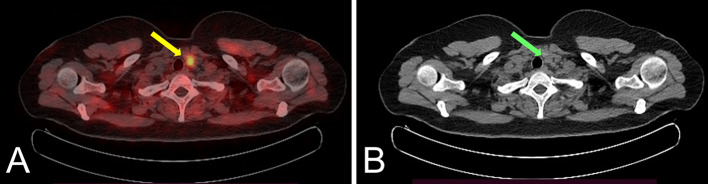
Axial [^18^F]fluorocholine PET/CT images (A) showing hyperfunctioning parathyroid in the middle-lower third of the left thyroid lobe (SUVmax 6.0 in the late phase) (yellow arrow) and CT coregistration (B) (green arrow)
